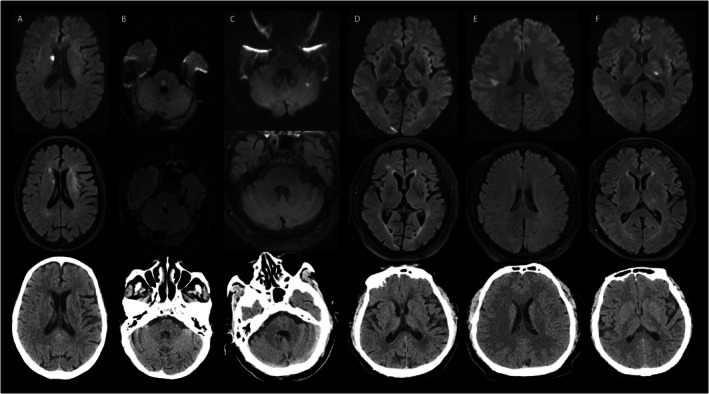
Distribution of ischemic stroke lesions in patients with isolated dizziness across different brain regions. This figure presents diffusion‐weighted imaging, fluid‐attenuated inversion recovery imaging, and computed tomography images of selected patients with isolated dizziness who underwent neuroemergency imaging. Panels A‐F correspond to individual patients.
